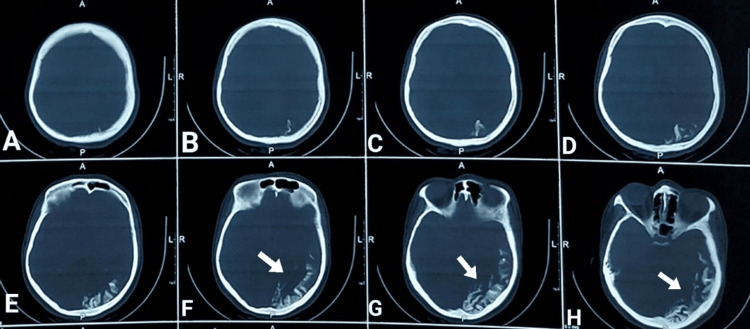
Axial non-contrast CT of the brain showing subcortical calcification in the left occipitoparietal region with a tram-track sign associated with parenchymal volume loss (prominent in images F, G, and H; pointed by white arrows).
